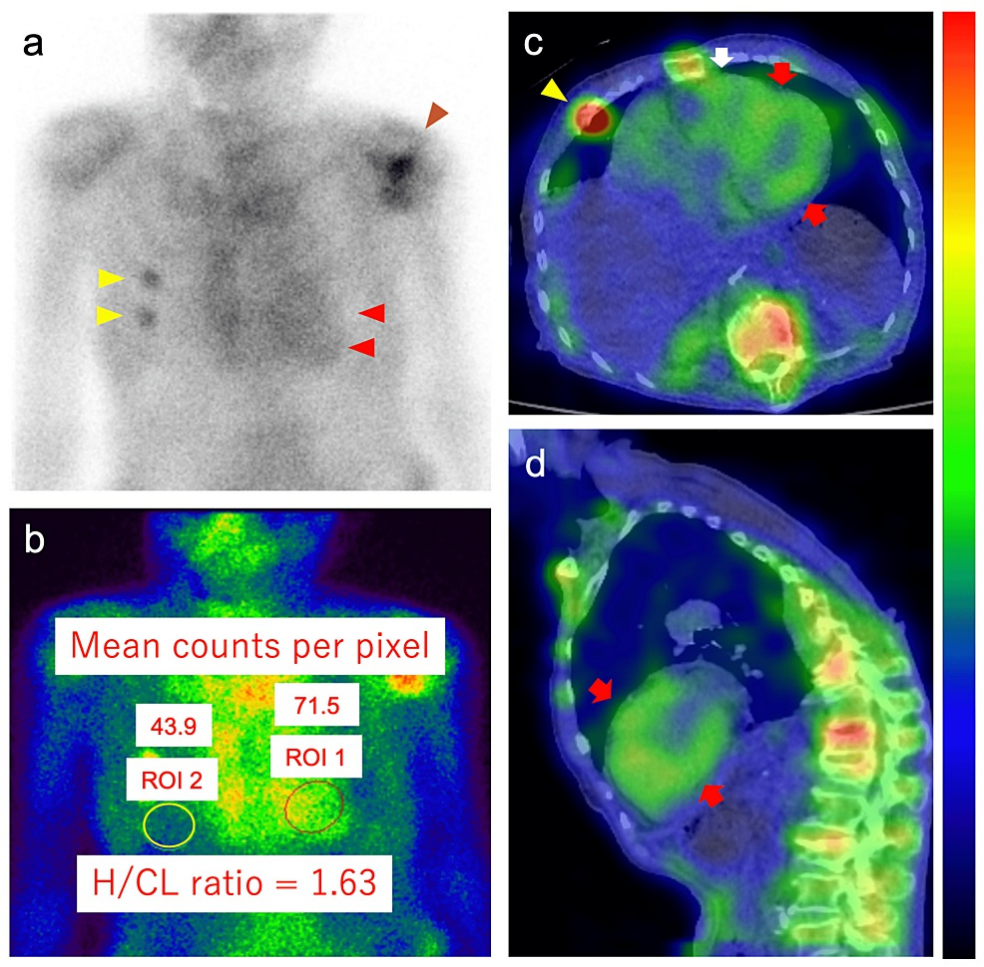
Technetium-99m-pyrophosphate scintigraphy images of Case 2 obtained 2 h after injection of radiotracers Technetium-99m-pyrophosphate planar imaging (anteroposterior view) shows Grade 3 semiquantitative visual scoring of cardiac retention (red arrowheads; high uptake greater than bone) (a) and heart-to-contralateral lung (H/CL) ratio on planar imaging of 1.63 (b). Single-photon emission computed tomographic images with fusion to computed tomographic images show tracer uptake in the left (red arrows) and right (white arrow) ventricular myocardium: (c) horizontal plane at the level of the heart and (d) sagittal plane. Regions of interest (ROIs) 1 and 2 indicate the ROIs positioned to maximize the coverage of the heart without including the adjacent lung and to minimize the overlap with the sternal or focal rib uptake, respectively. Yellow arrowheads and the brown arrowhead indicate the tracer uptake in the right ribs and left shoulder, respectively. Kyphosis is also shown.
